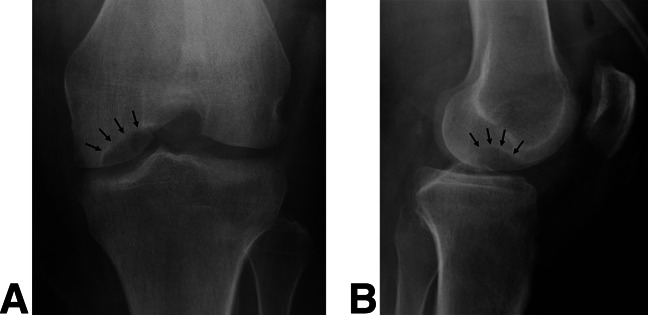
A and B, Orthogonal plain radiographs of the right knee demonstrating a corresponding, well-circumscribed lucency in the vicinity of the osteochondral lesion (arrows).
